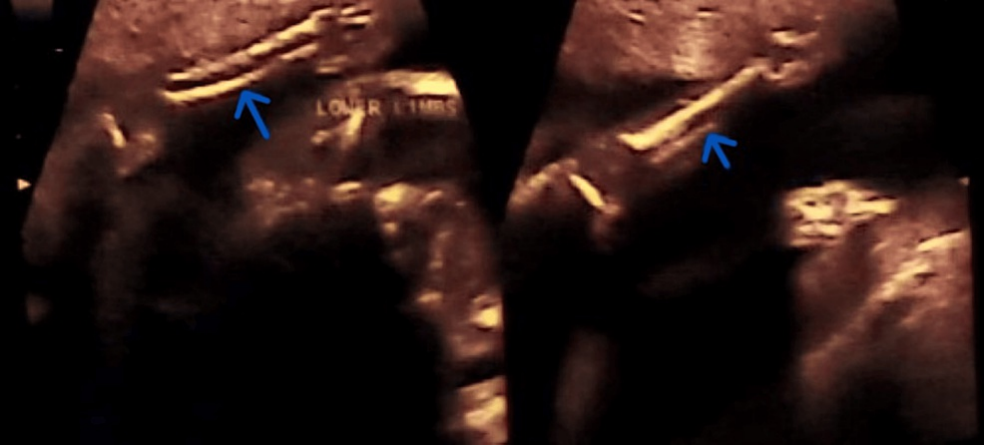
Maternal ultrasonography in the second trimester showing a fixed position of the legs with persistent knee extension (indicated by an arrow).
